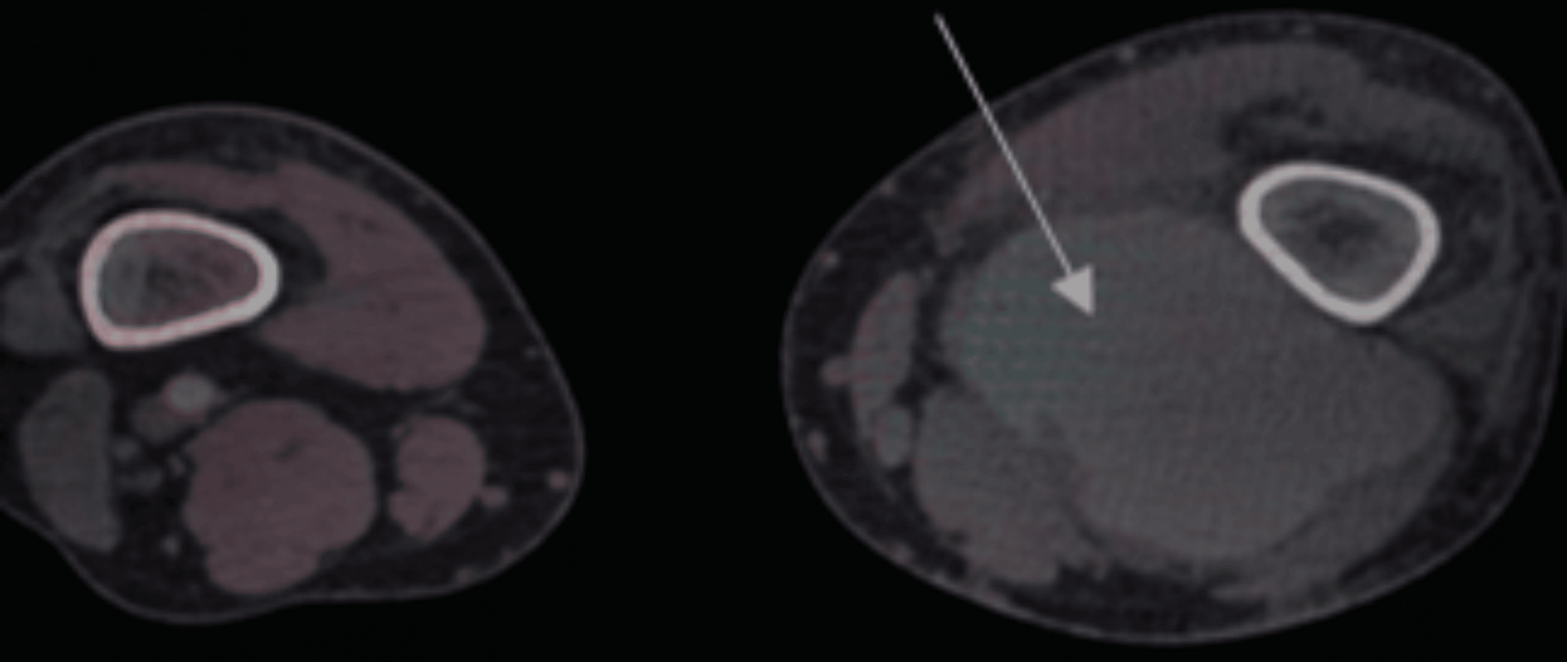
CT angiography of the lower limbs showing a ruptured left popliteal aneurysm. The arrow indicates the ruptured aneurysm.
